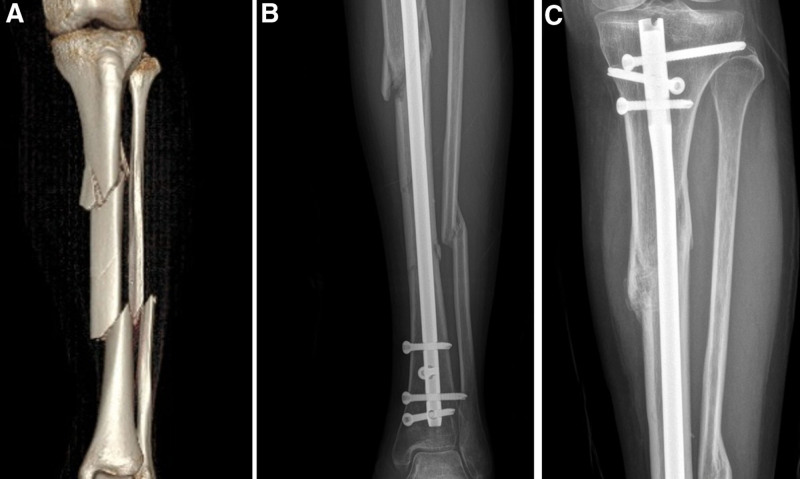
(A) A 49-year-old female patient involved in a traffic accident with STF. (B) Six months after internal fixation with the IMN, X-ray revealed nonunion. (c) Three months after iliac crest bone grafting, X-ray revealed union. IMN = intramedullary nail, STF = segmental tibia fractures.
